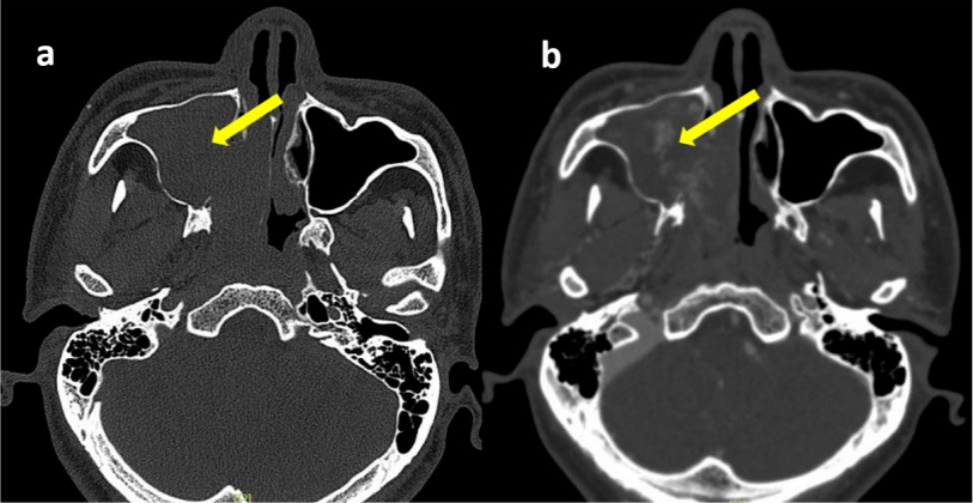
CT scan of the paranasal sinus (2020-01-08) (a). Enhanced CT scan of the paranasal sinus (2020-01-15) (b). The tumour occupied part of the right maxillary sinus and nasal cavity (arrow)
